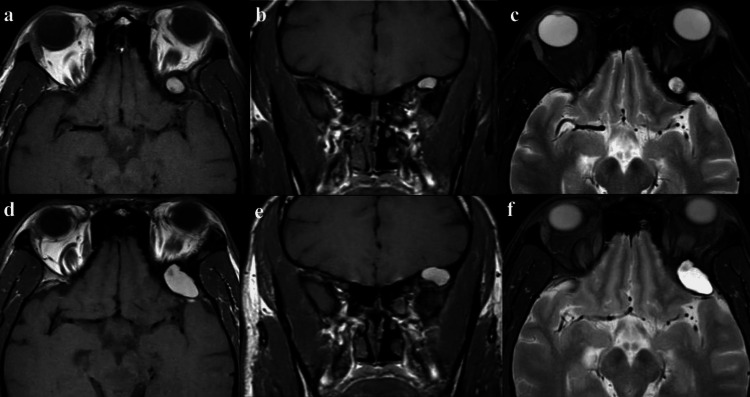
Left sphenoid wing lesion with mass effect (a, b) Axial and coronal T1-weighted MR images showing an 11 x 7 mm expansile, heterogeneous, hyperintense lesion in the left greater wing of the sphenoid, causing mass effect in the left orbital content. (c) Axial T2-weighted MR image showing hyperintense signal changes. (d-f) Axial and coronal T1-weighted MR images and axial T2-weighted MR demonstrating interval growth in the lesion to 24 x 15 mm over six years.
